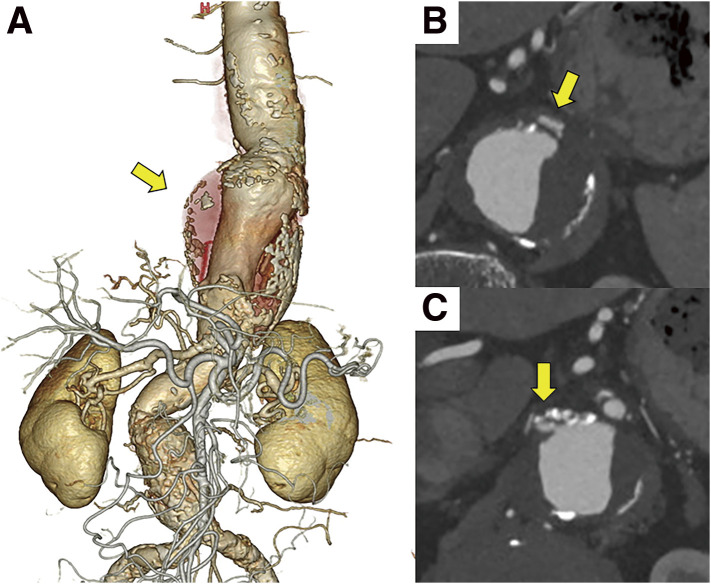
Preoperative contrast-enhanced CT. (A) Thoracoabdominal aneurysm (yellow arrow) in 3D CT angiography. (B) Celiac artery stenosis (yellow arrow). (C) Right renal artery stenosis (yellow arrow). CT: computed tomography
